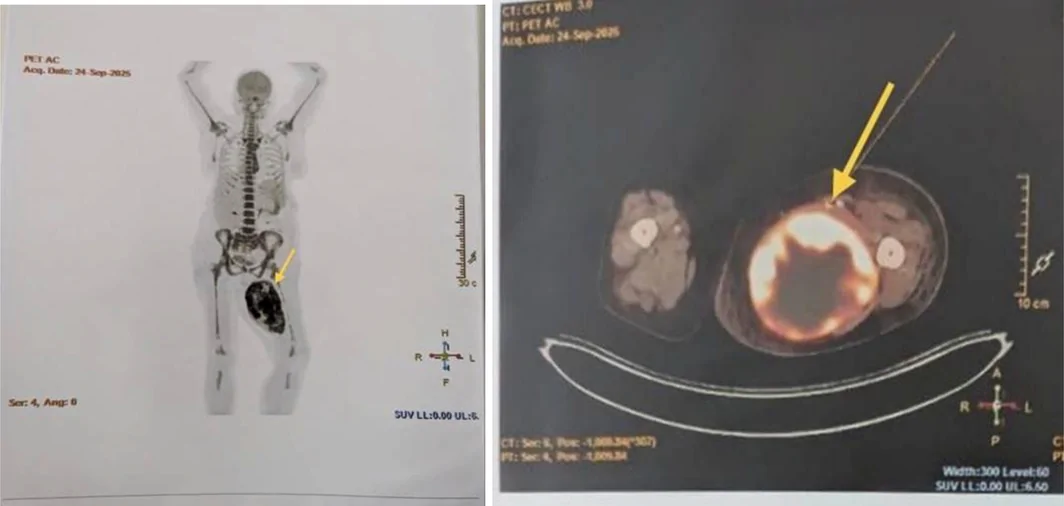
PET CT scan showing heterogenous density mass with peripheral FDG uptake having central necrotic area involving muscles of medial compartment of left thigh; consistent with biopsy proven sarcoma.
